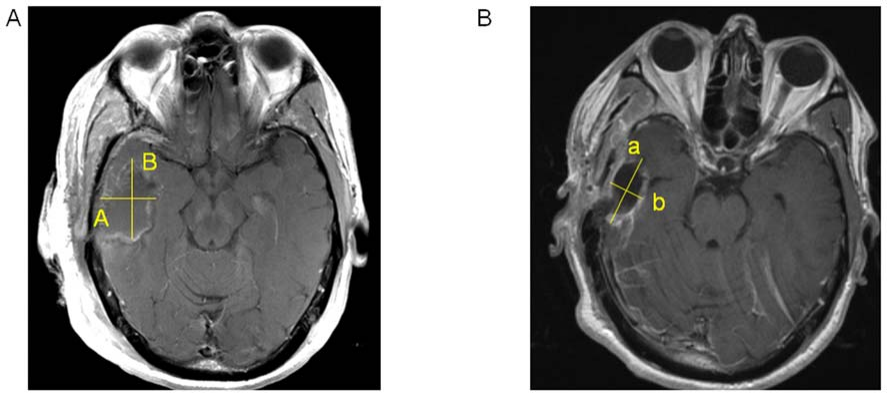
Detection of Enhancing Tumor Volume Despite Resection Cavity Collapse. A) T1-weighted post-contrast axial image showing a resection cavity with rim enhancement. RECIST measurement would be A and Macdonald measurement would be “A * B”. B) T1-weighted post-contrast axial image showing the same patient 3 months postoperatively who had collapse of his resection cavity. RECIST measurement would be “a” and Macdonald measurement would be “a * b”, both of which would be smaller than the measurements from the initial scan above, but this change would be describing only the resection cavity configuration and not the underlying tumor burden.
